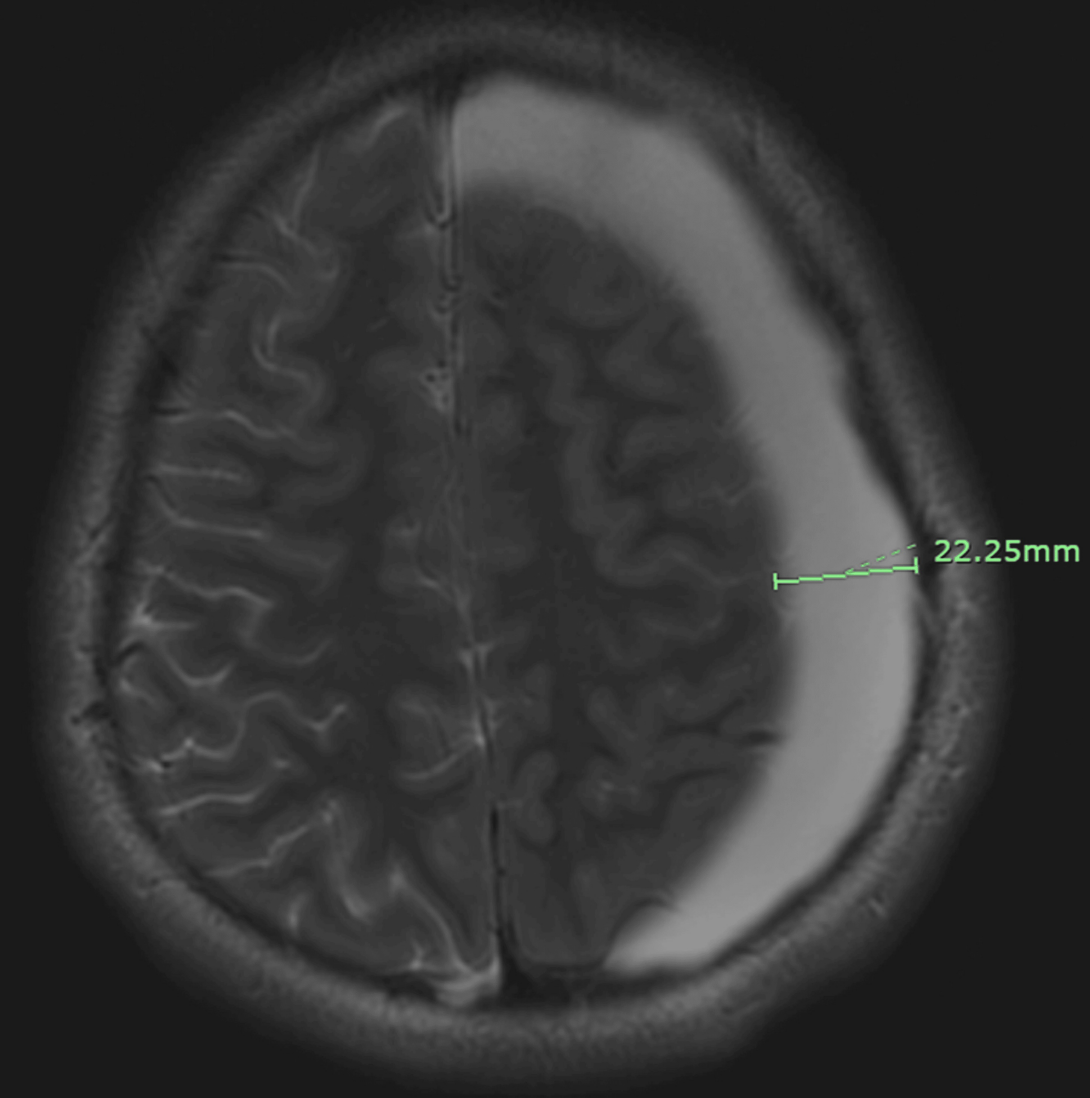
Axial T2-weighted brain MRI without contrast using the PROPELLER technique demonstrating 22.25 mm left temporal arachnoid cyst causing sulcal effacement. PROPELLER technique: Periodically Rotated Overlapping Parallel Lines with Enhanced Reconstruction
